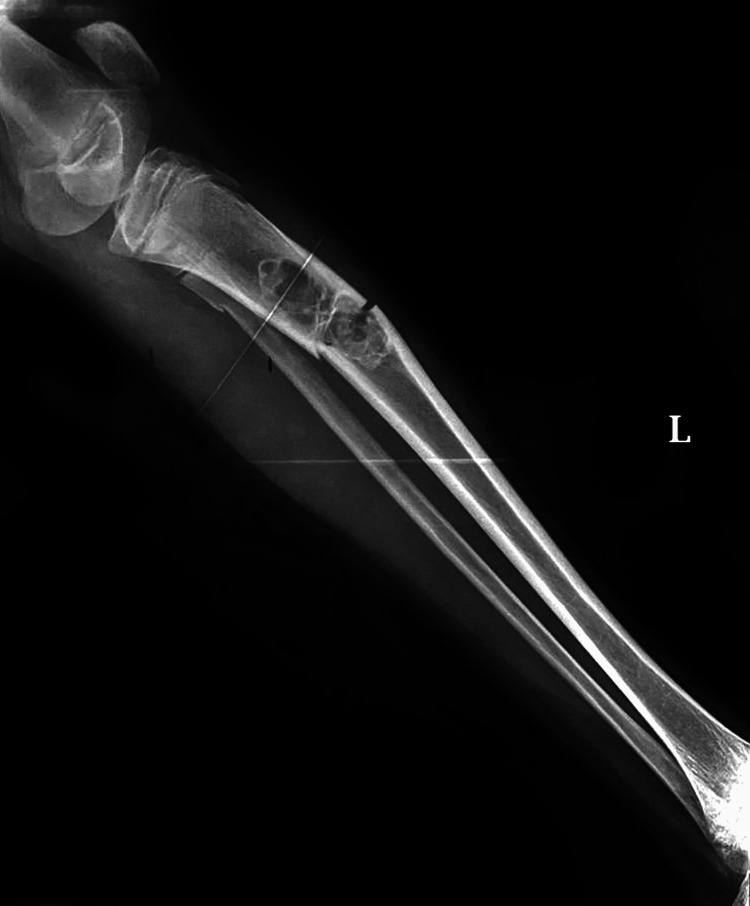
Non-contrast computed tomography scan of left leg showing lytic lesion, eccentric, and soap bubble appearance growth involving metaphysis of the tibia
